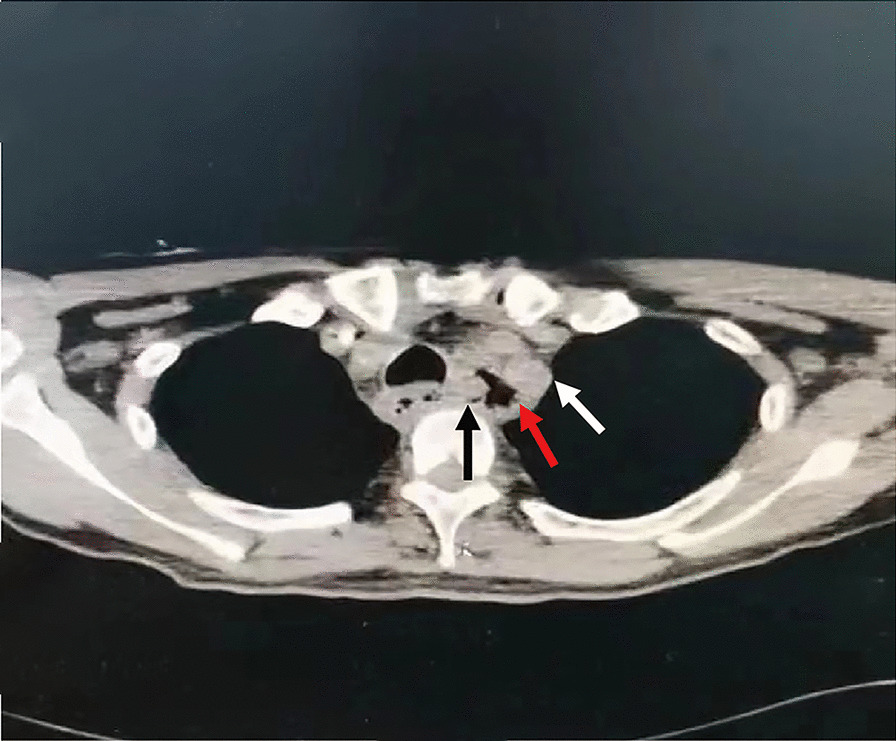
Image from CT scan. CT showed that the local soft tissue was thickened in the esophagus at the cervicothoracic junction, and a gaseous cavity was present on the left posterior wall of the esophagus (red arrow), approaching the left subclavian artery (white arrow). Esophageal breach was detected (black arrow)
